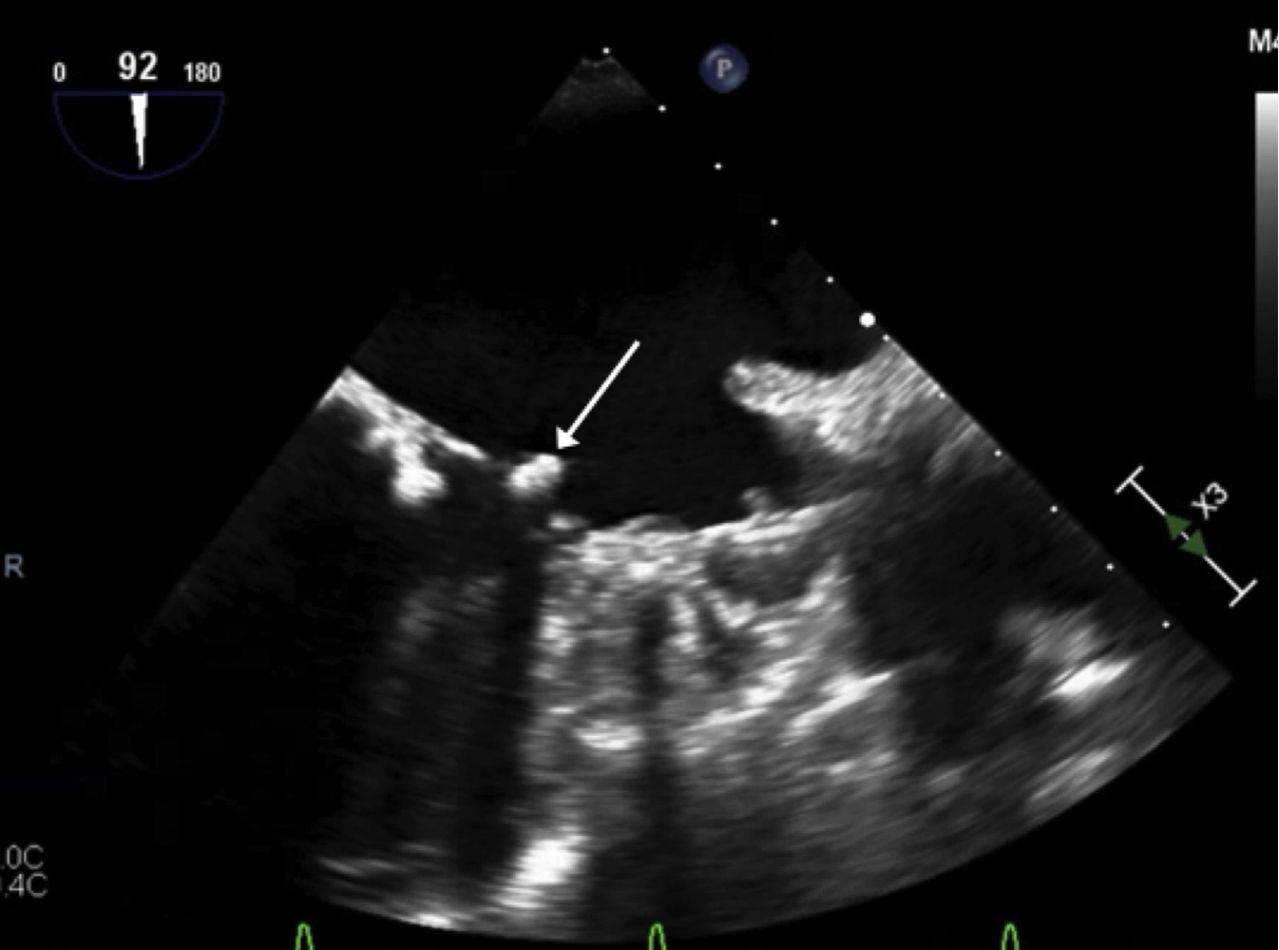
Intraoperative image of mitral valve mass adjacent to the WATCHMAN (Boston Scientific, Marlborough, MA) device. Transesophageal echocardiogram image at 90^o^ demonstrating the proximity of a mitral valve mass (*white arrow*) to the deployed WATCHMAN device.
